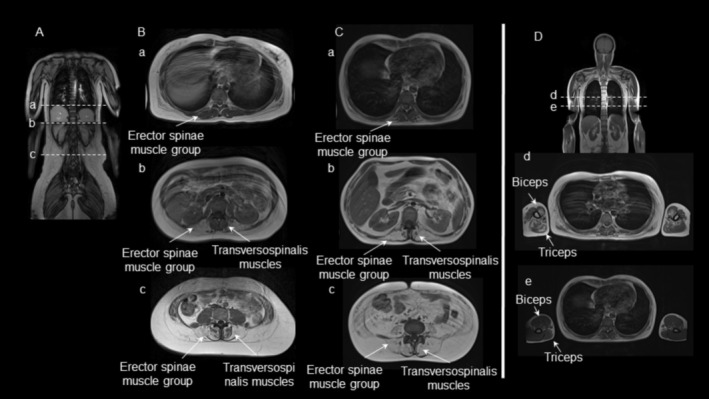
Fat replacement gradient in paraspinal and arm muscles. (A, D) Localizer images. Coronal view of WB‐MRI, displaying the corresponding axial slices (a–e). (B) P33, LGMDR3, and (C) P20, LGMDR4: Showing severe involvement of erector spinae at the lumbar level with sparing of the upper thoracic part. Transversospinalis muscles also show a similar gradient. (D) P20, LGMDR4: A proximo‐distal gradient of involvement is evident in biceps and triceps brachii muscles.
